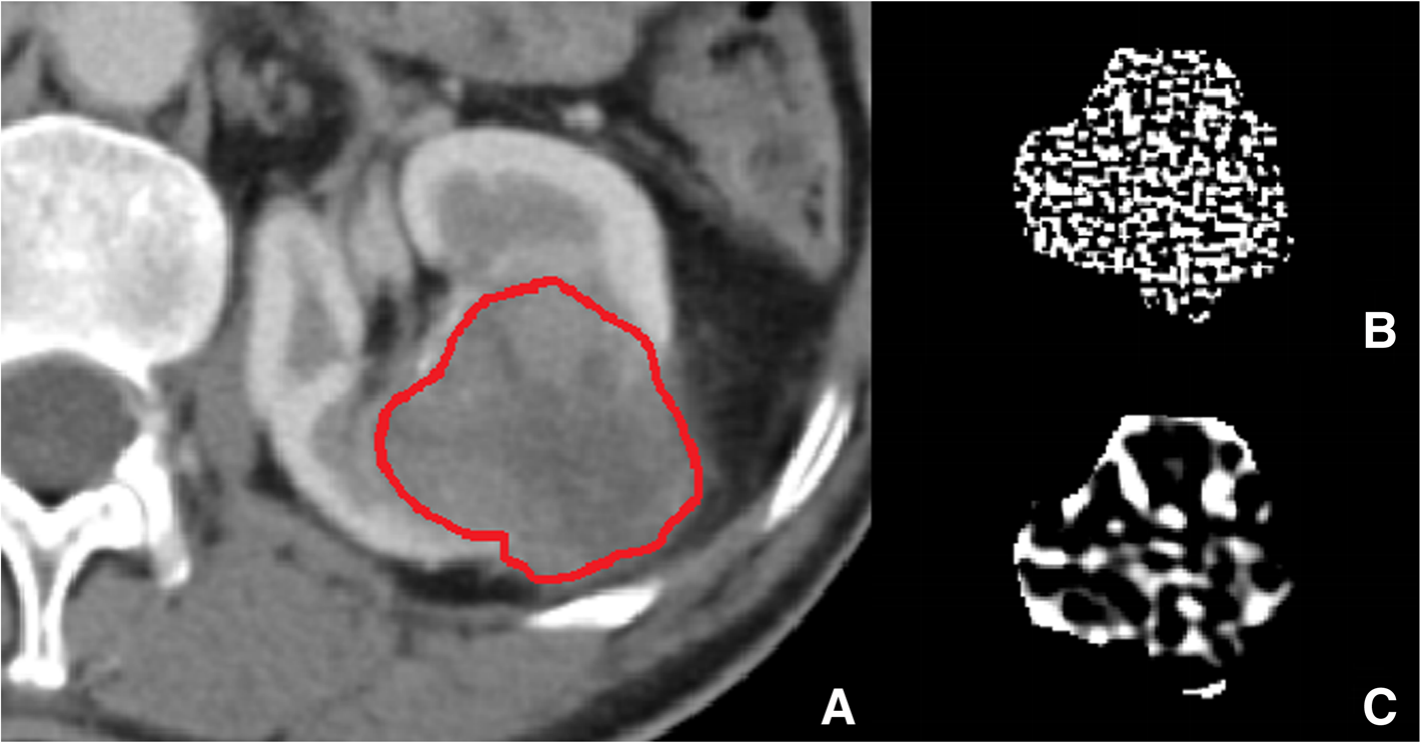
CT texture analysis to evaluate a clear-cell renal cell carcinoma(ccRCC). a Axial CT images of ccRCC texture feature before filtering, ROI was created within the confines of the tumor for texture analysis. b ccRCC texture feature after fine-scale filtering. c ccRCC texture feature after coarse-scale filtering
